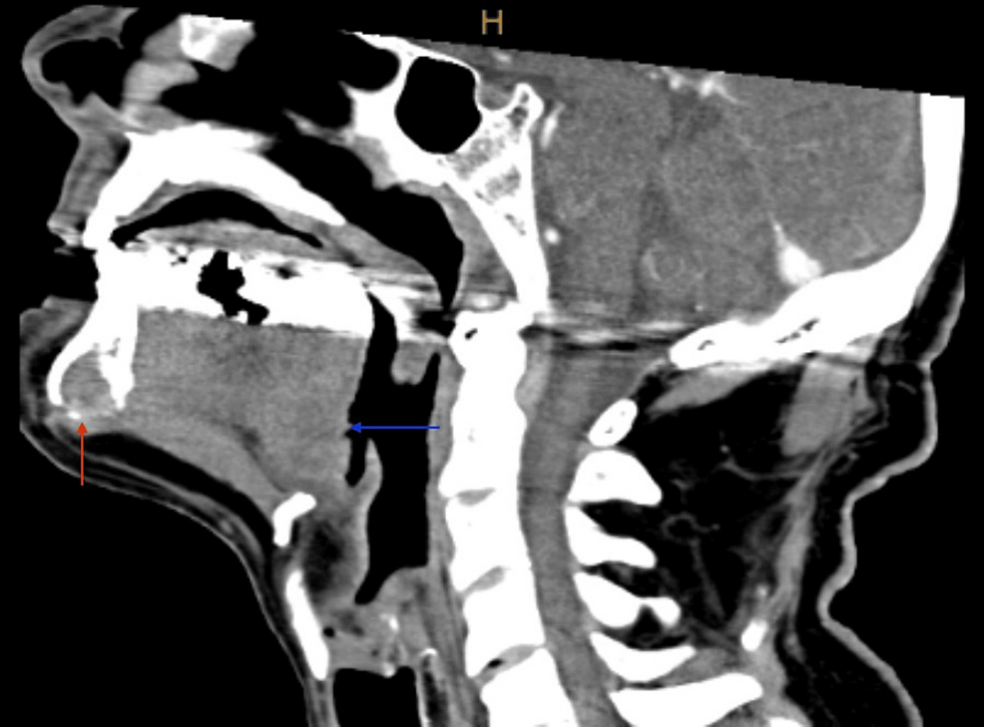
Sagittal CT Neck of Mandibular and Base of Tongue Mass Sagittal CT neck with contrast showing a soft tissue mass in the mandibular symphysis (red arrow) and a left base of tongue mass (blue arrow)
